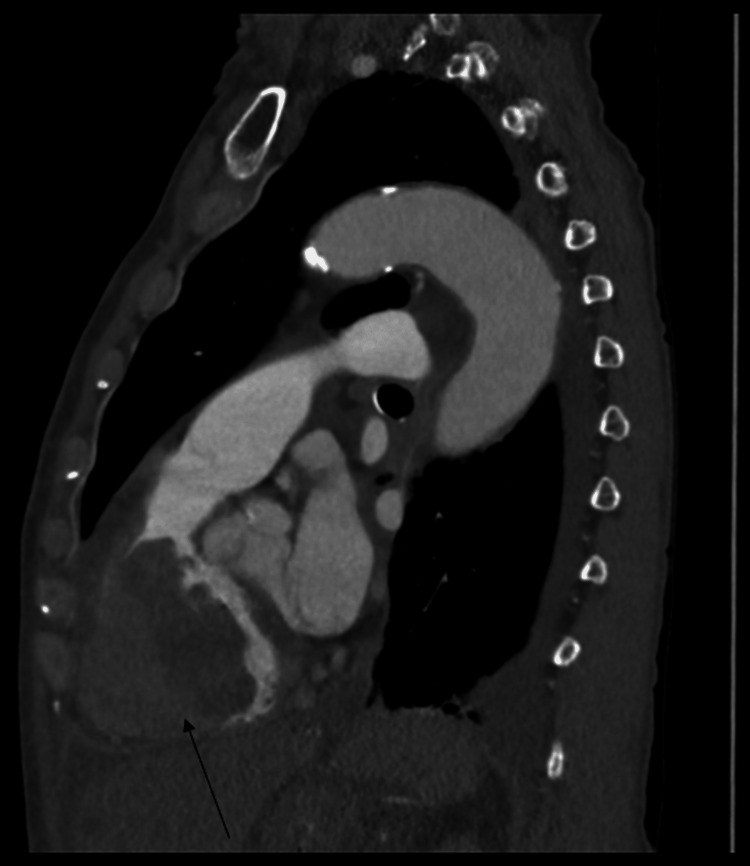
CT chest with contrast in the sagittal plane showing a large intracardiac mass within the right ventricle (black arrow).
